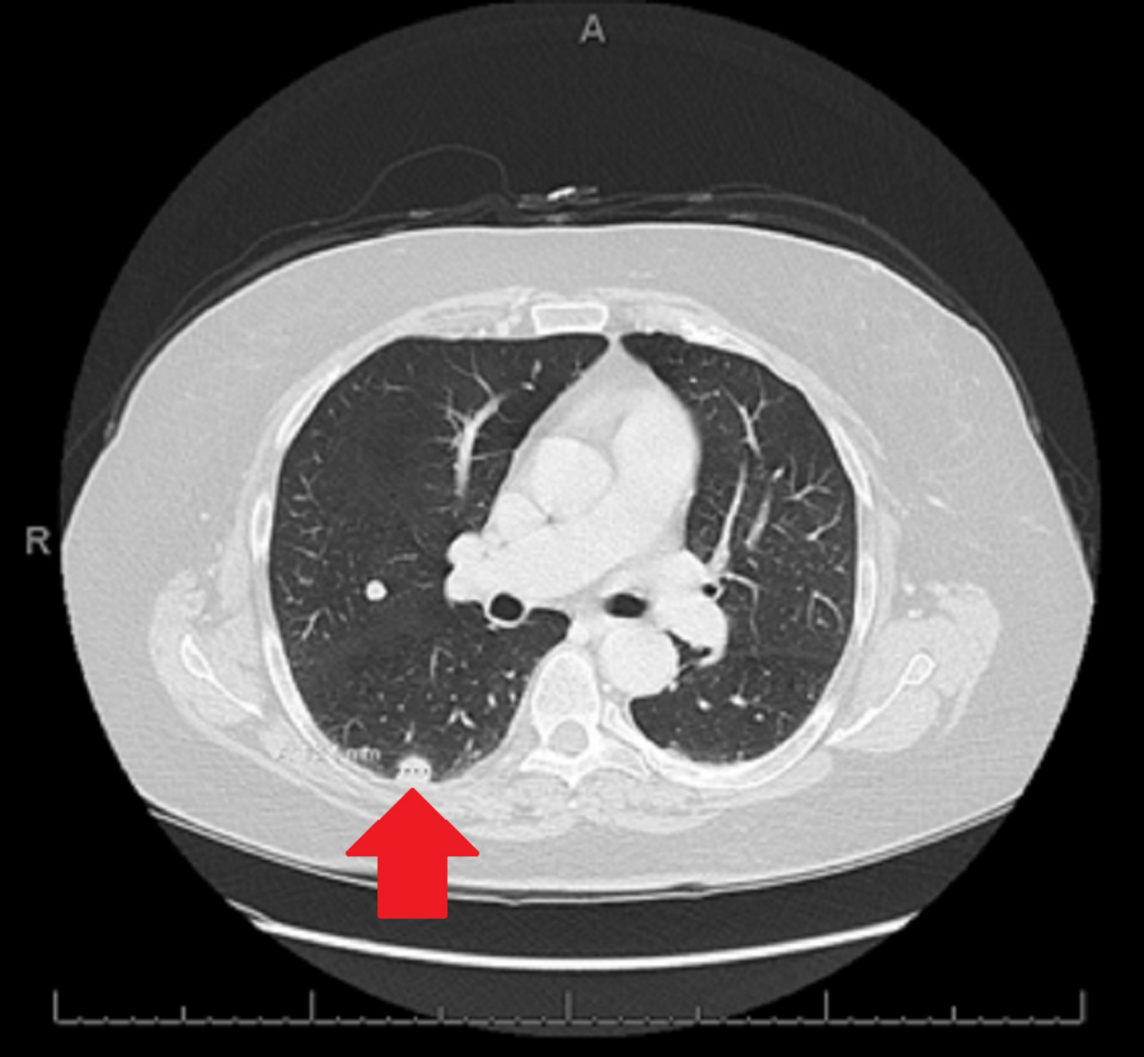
Pulmonary nodule via CT scan Interval enlargement of pulmonary nodules; the example here is the superior segment of right lower lobe (previously 0.6 cm, now 1.3 cm).
